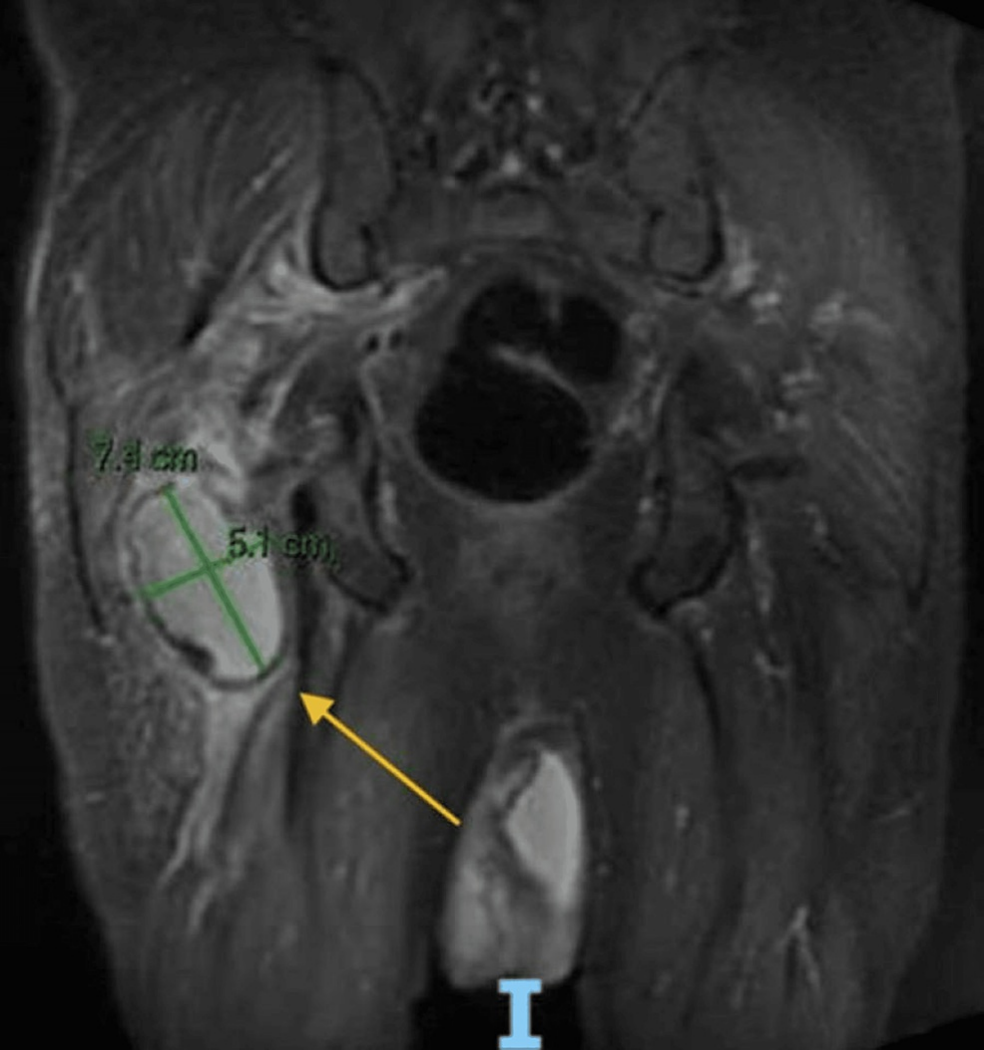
MRI pelvis MRI showing bilateral periprosthetic osteolysis is worse on the right side. A pseudotumor is noted on the right side, exerting a mass effect on the sciatic nerve at the level of the ischial tuberosity.
